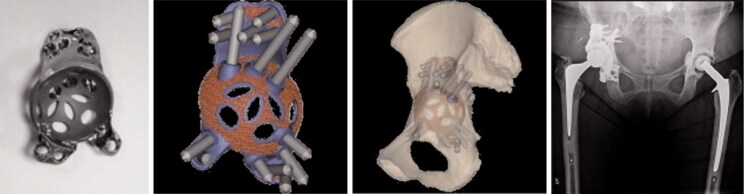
Custom made impalnt, planned screw positions and implant position based on CT scan. CT was taken prior to surgery. The radiograph shows the postoperative result. The position of the implant after surgery was compared with the preoperative planning using the pre- and postoperative CT scans.
